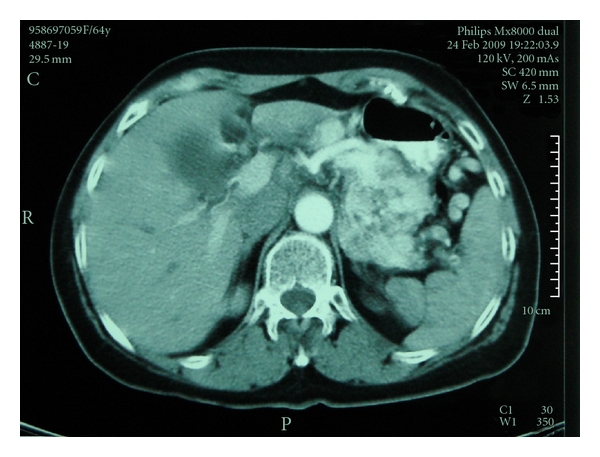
Contrast-enhanced axial CT scan through the pancreas shows a large hypervascular mass in the pancreatic body and tail. Note the presence of dilated perisplenic vessels.
